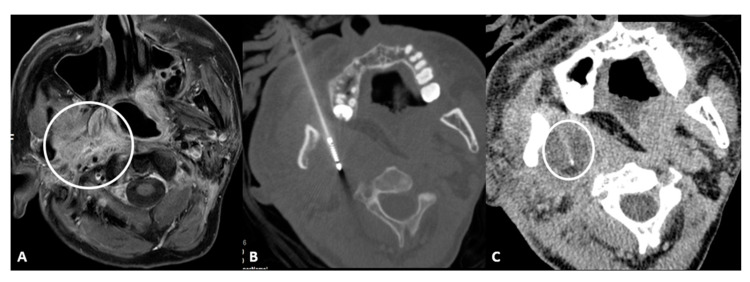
56-year-old male with neuropathic pain due to right oropharyngeal squamocellular carcinoma recurrence after tracheostomy, neck lymph node dissection, mandibulotomy, right oropharyngeal resection with free anterolateral thigh flap. (A) T1-weighted postcontrast axial MRI scan showing malignant involvement of trigeminal nerve (circle). (B) Axial CT scan showing cryoablation needle placement. (C) Intraprocedural axial CT scan showing ice ball formation around the needle (circle).
